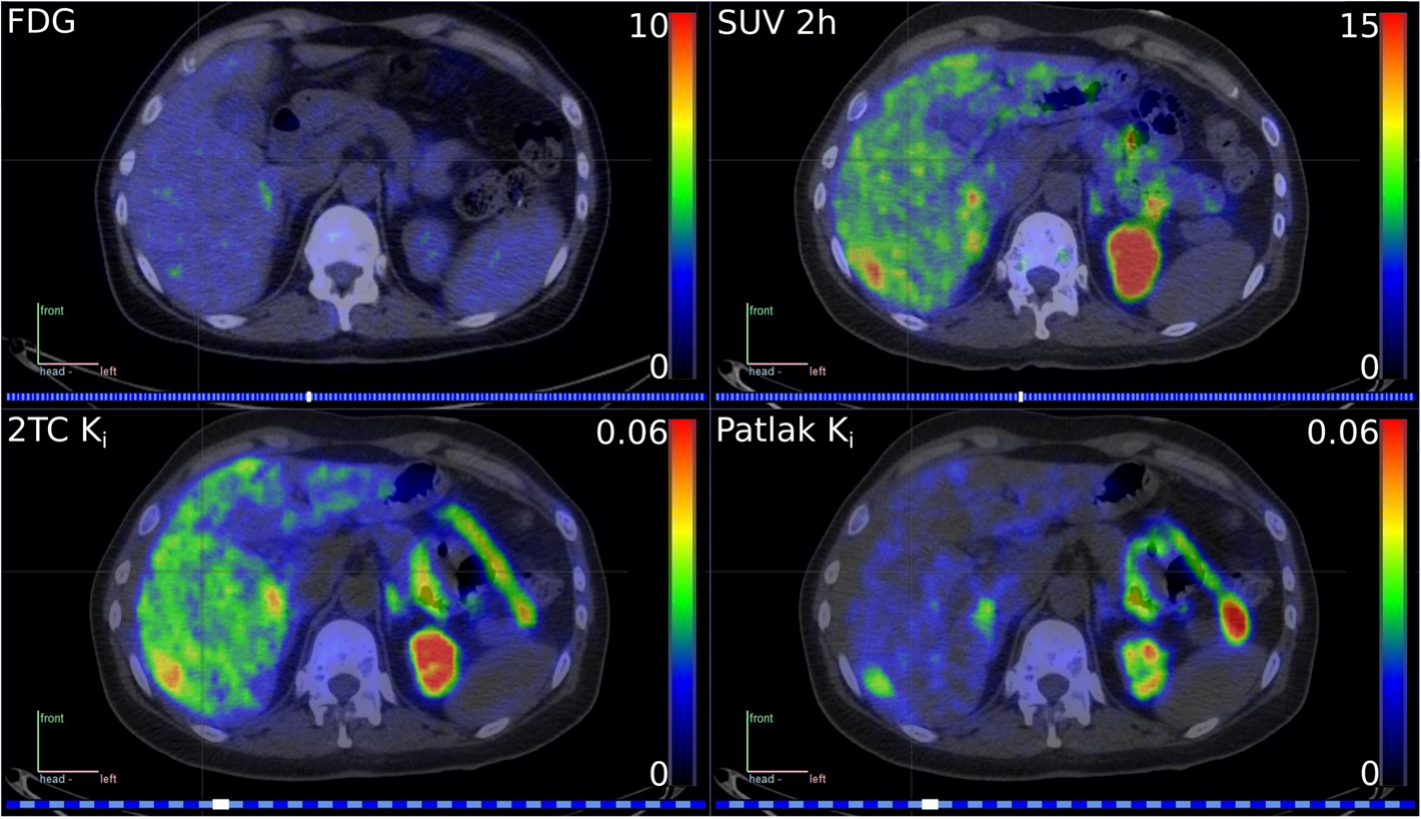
FDG PET and ABY-025 PET SUV 2 h and parametric images of both Patlak *K*_*i*_ and 2TC-3k *K*_*i*_ in a breast cancer patient with multiple small liver metastases
